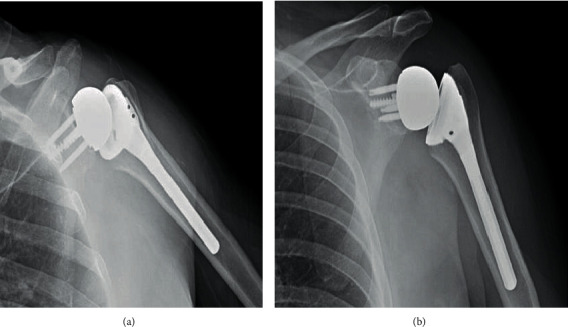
(a, b) Postoperative radiographs of Case 1 demonstrating reverse shoulder arthroplasty following fixation of large glenoid rim fracture via the baseplate.
